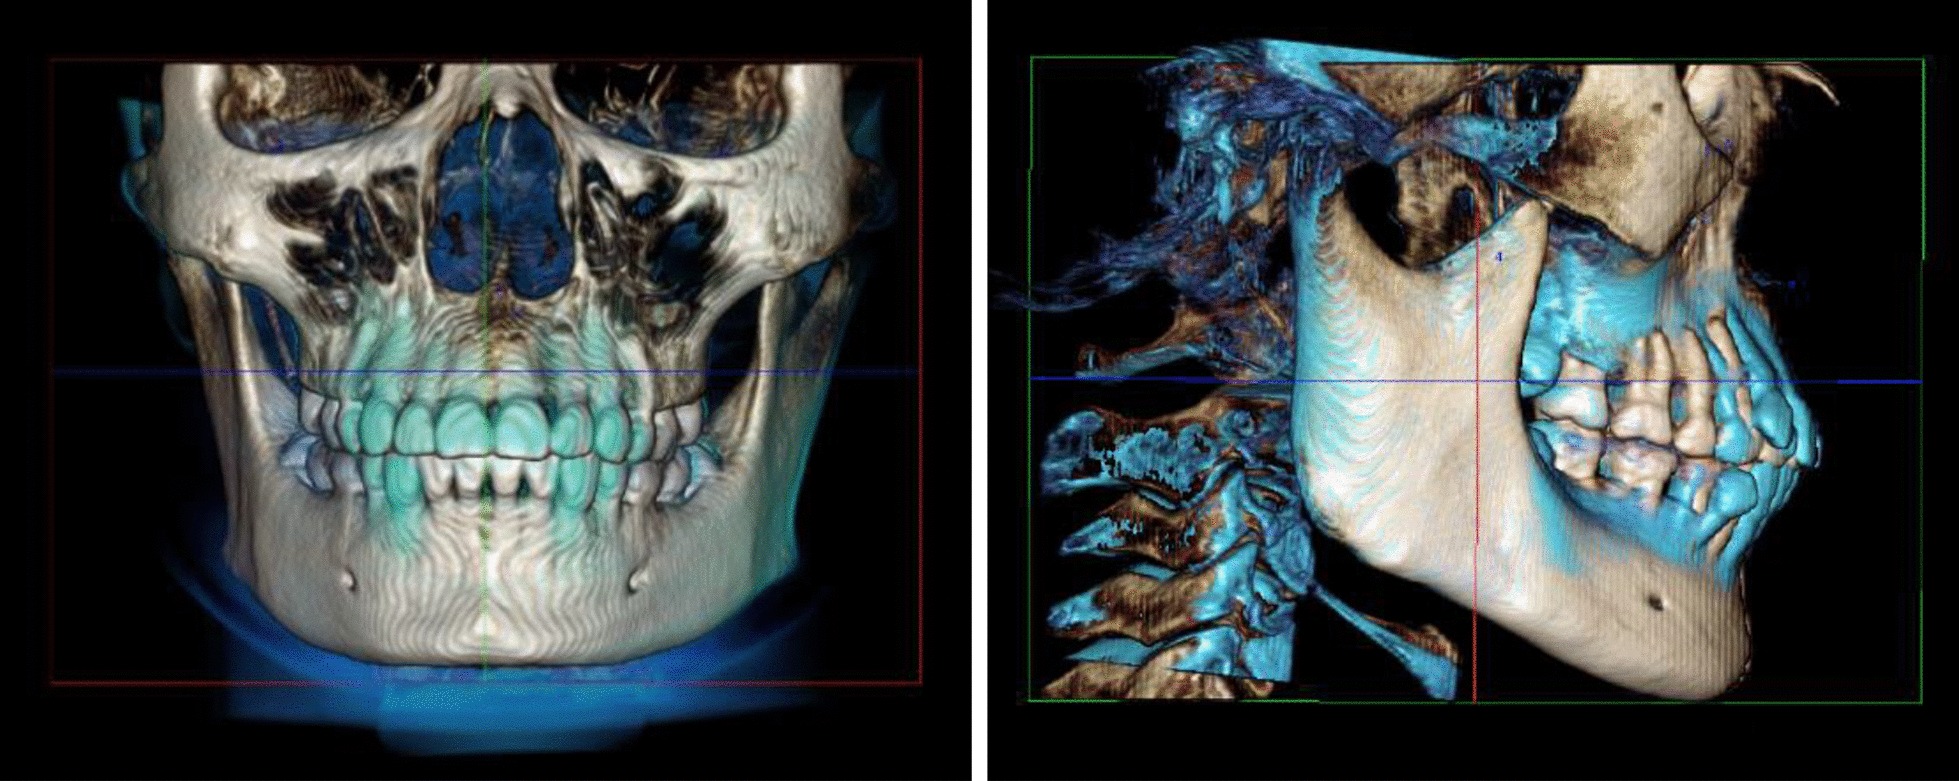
Landmark CBCT superimposition profiles. The same 4 landmark were included: ANS, PNS, bilateral orbitales
